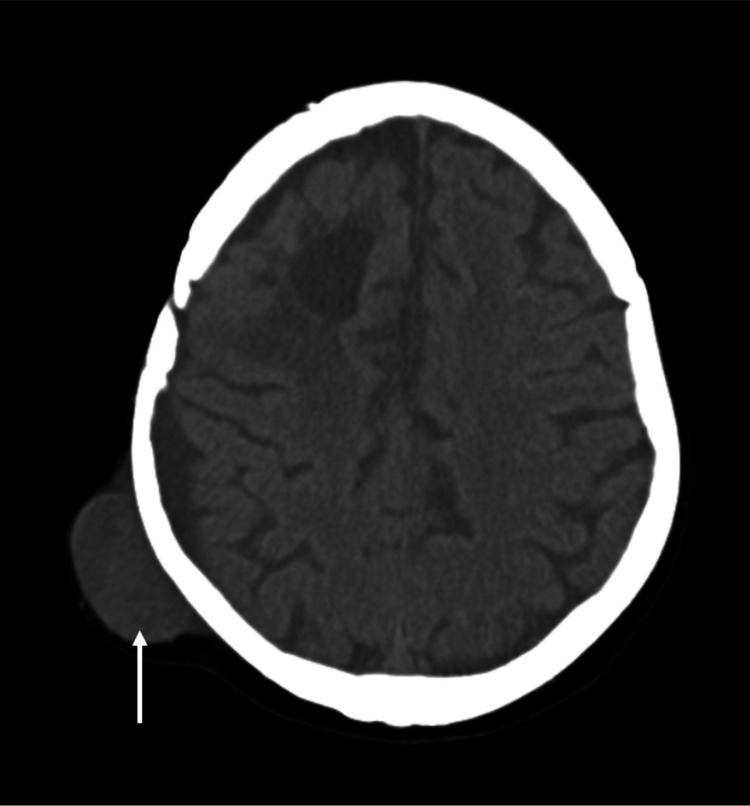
Brain CT with right parietal epicranial expansive lesion.
